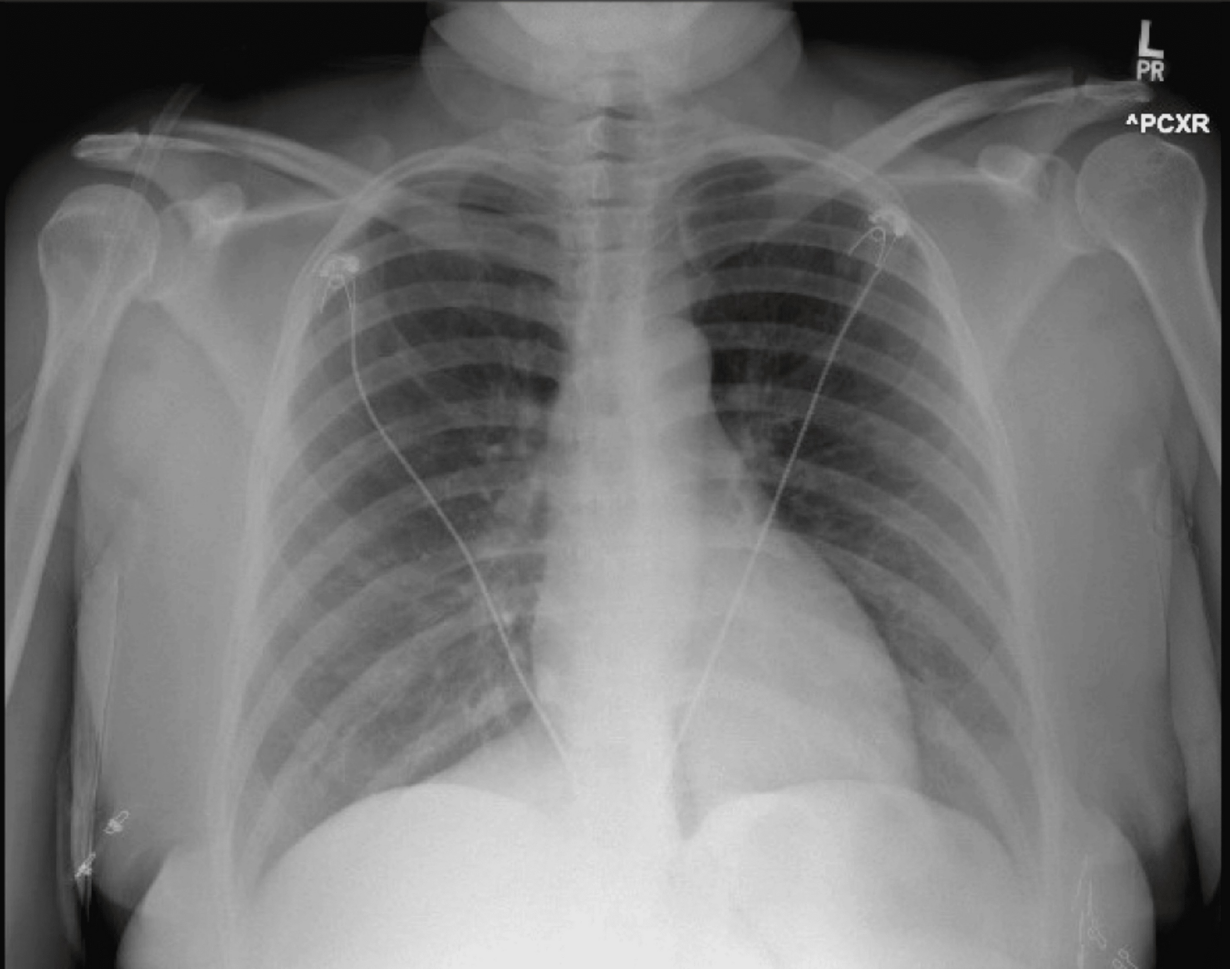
Coronal chest X-ray on presentation
